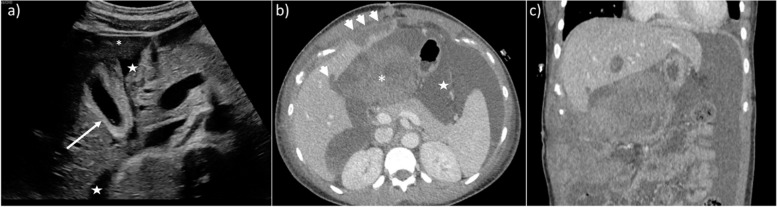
Hepatic rupture in a 27-year-old woman around childbirth. The patient complained of severe right upper quadrant and scapular pain. Alanine aminotransferase and aspartate aminotransferase were augmented, prothrombin time was 30%. Transverse abdominal US scan (a) shows the presence of hypoechoic areas (*), free fluid around the liver (stars), and striated thickening of the gallbladder walls (arrow), raising suspicion for pre-eclampsia. Axial (b) and coronal (c) CT scan on portal venous phase (b) demonstrate hepatic lacerations (arrowhead) with surrounding hemoperitoneum and a large peritoneal hematoma (*). Patient underwent emergency cesarean section and liver transplant
